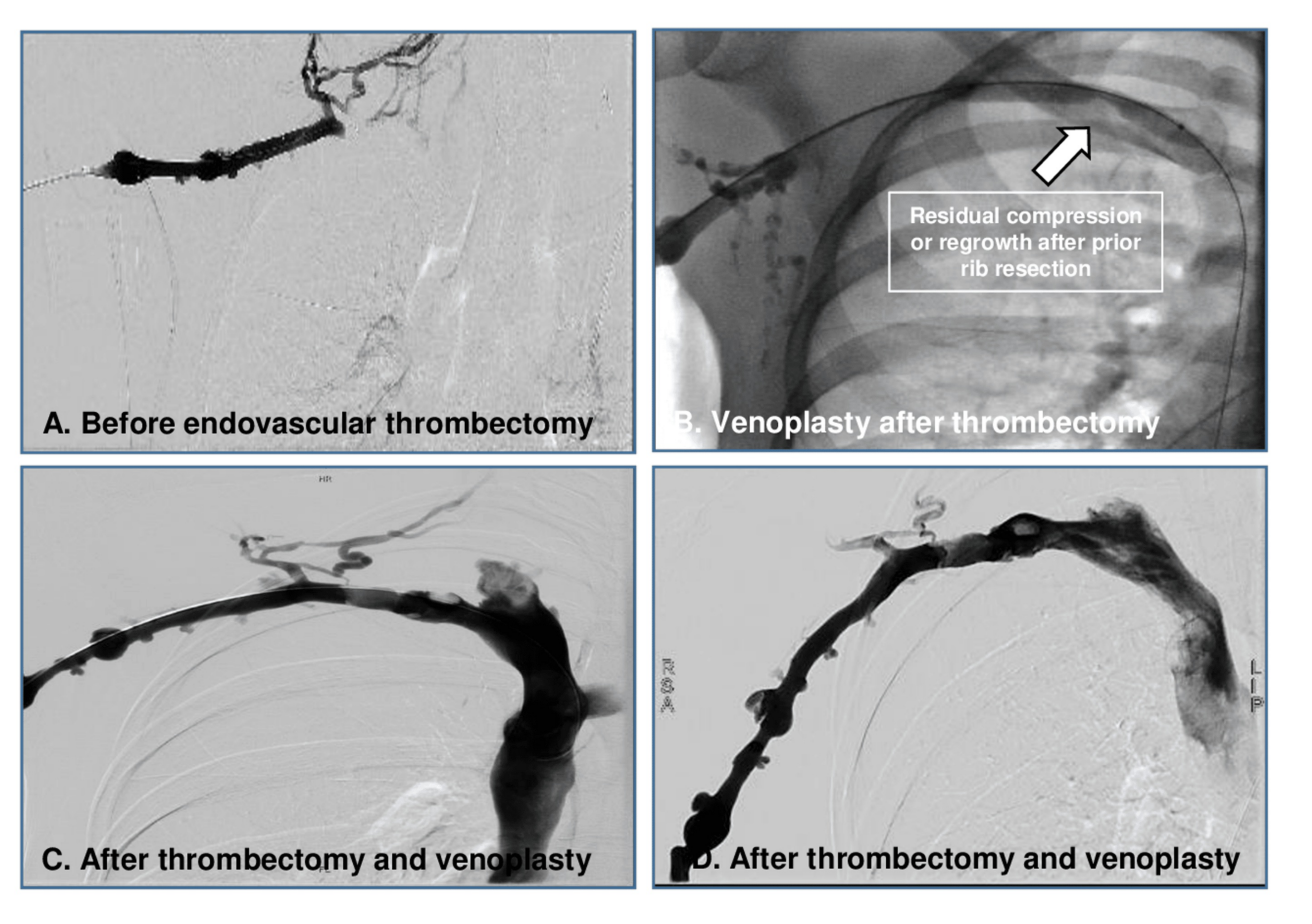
MRI angiography and duplex sonography before and after thrombectomy A) Residual compression or regrowth after prior rib resection; B) Before endovascular thrombectomy; C) After endovascular thrombectomy; D) After endovascular thrombectomy
